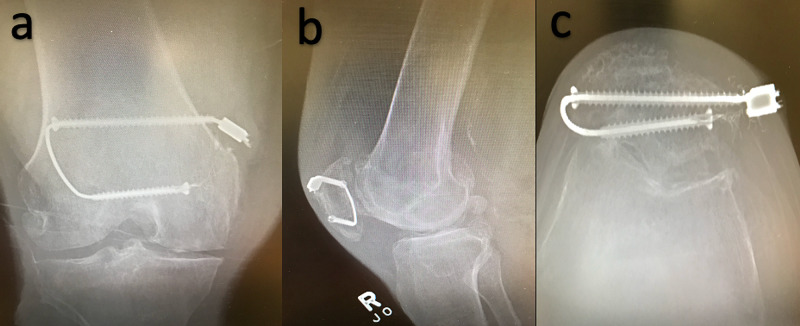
X-ray views on presentation Anteroposterior (AP) (a), lateral (b), and sunrise (c) X-ray views of the right knee demonstrated two horizontally oriented cannulated screws traversed by metal cable with segment of cable abutting the skin over the superomedial knee. The superior screw appears with step off medial bone surface.
